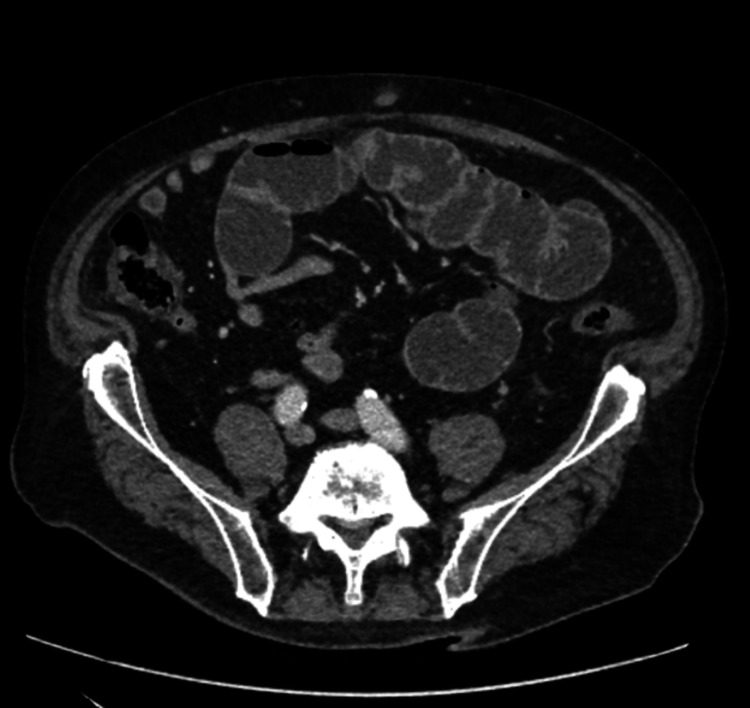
Computed tomography scan on admission showing the distended small bowel and transition zone in the retro-umbilical region.
